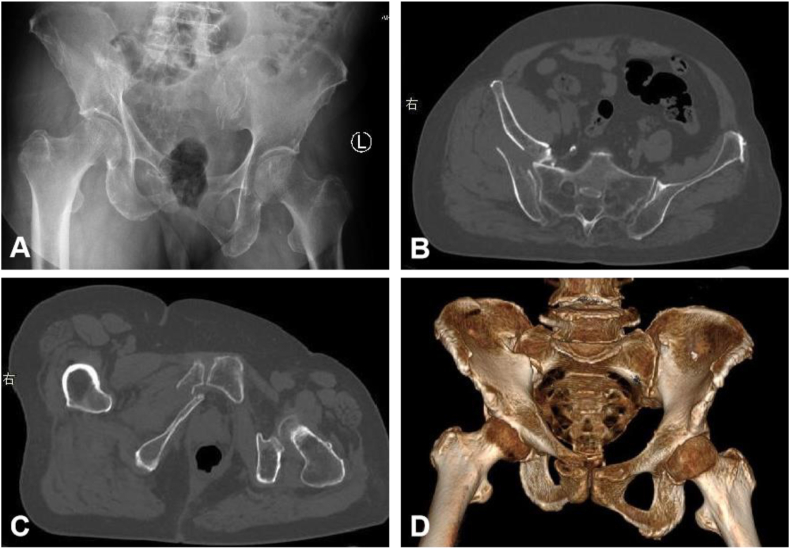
Image of the displaced pelvic fracture in a 83-year-old female with heart disease and severe osteoporosis (A: anterioposterior view; B–C: axial CT view of fractured posterior and anterior ring; D: 3D reconstruction view).
